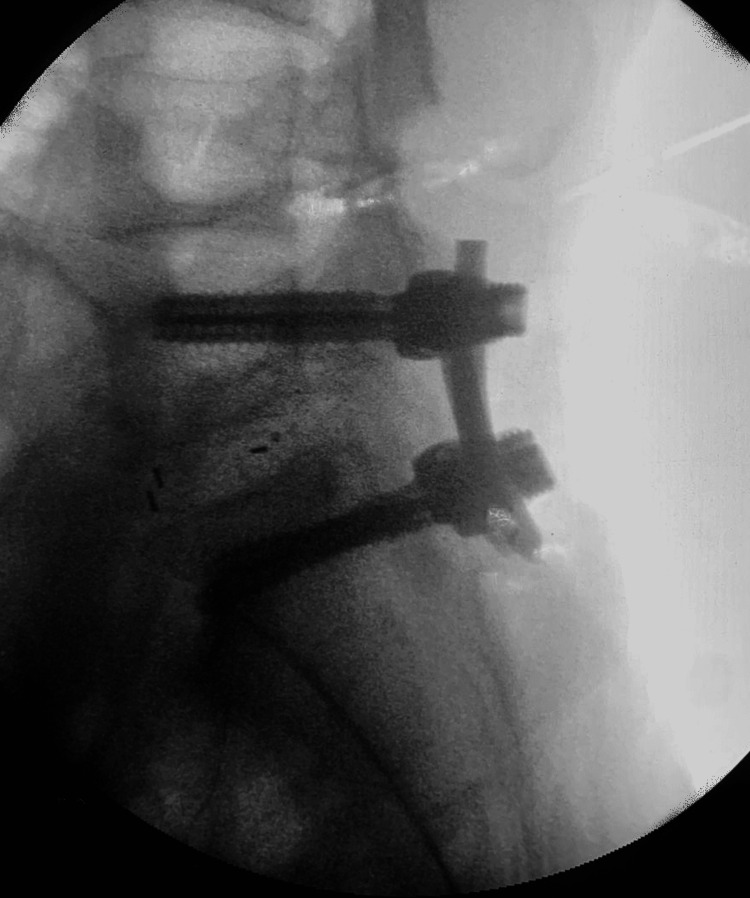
Immediate post-operative lateral view radiograph showing revision of spinal construct after removal of TM cage and insertion of TLIF PEEK cage.
